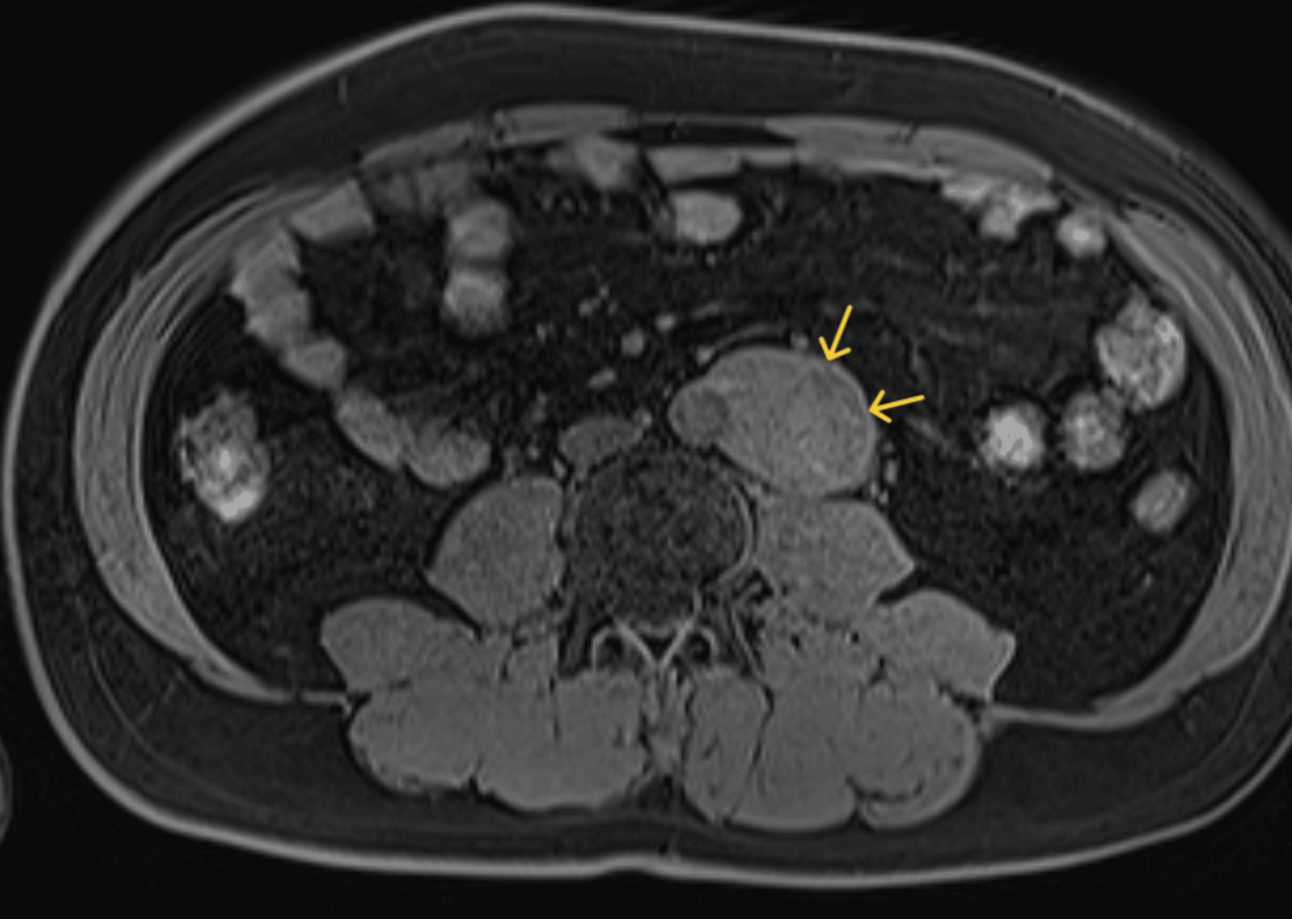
MRI T1-enhanced image The solitary fibrous tumor (arrows) was seen tangential to the left side of the abdominal aorta, and there was no interposition of fatty layer between the abdominal aorta and the SFT MRI, magnetic resonance imaging; SFT, solitary fibrous tumor
